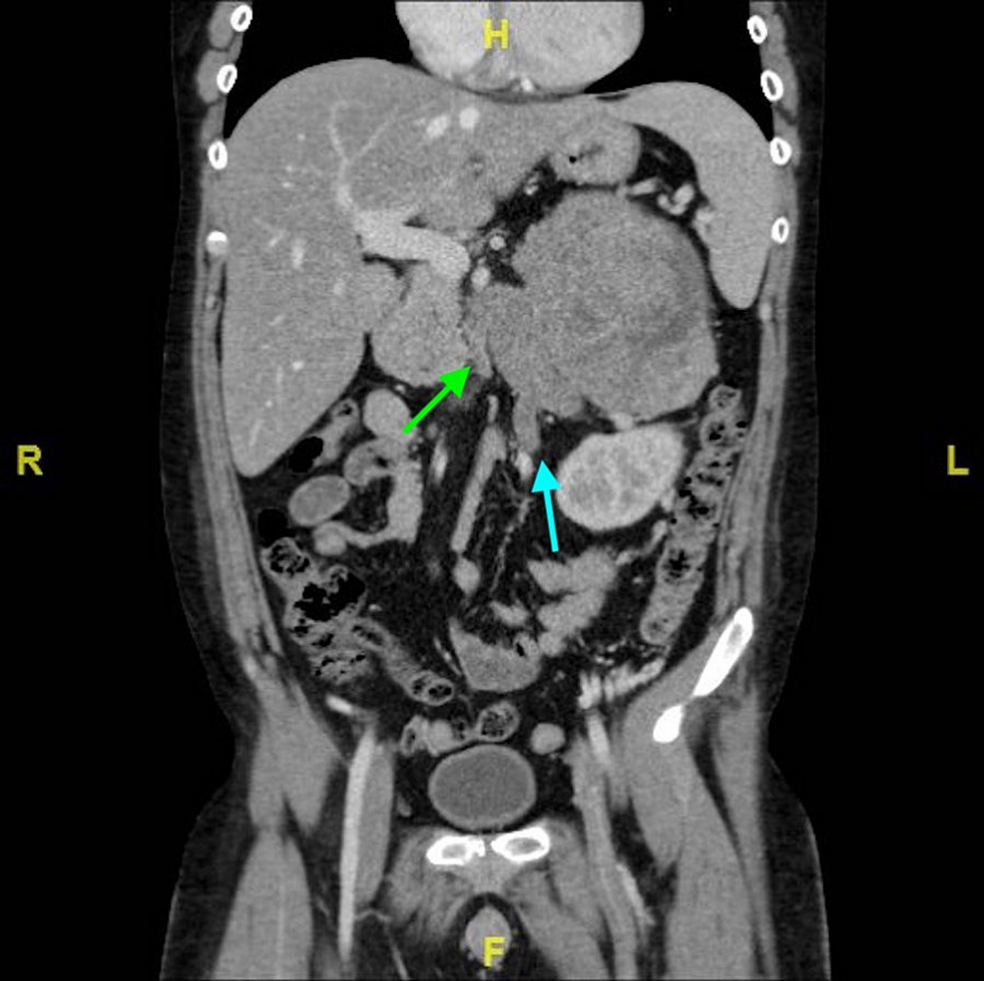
CT scan showing tumor thrombus extending into a left renal vein (green arrow) and left gonadal vein (blue arrow).
